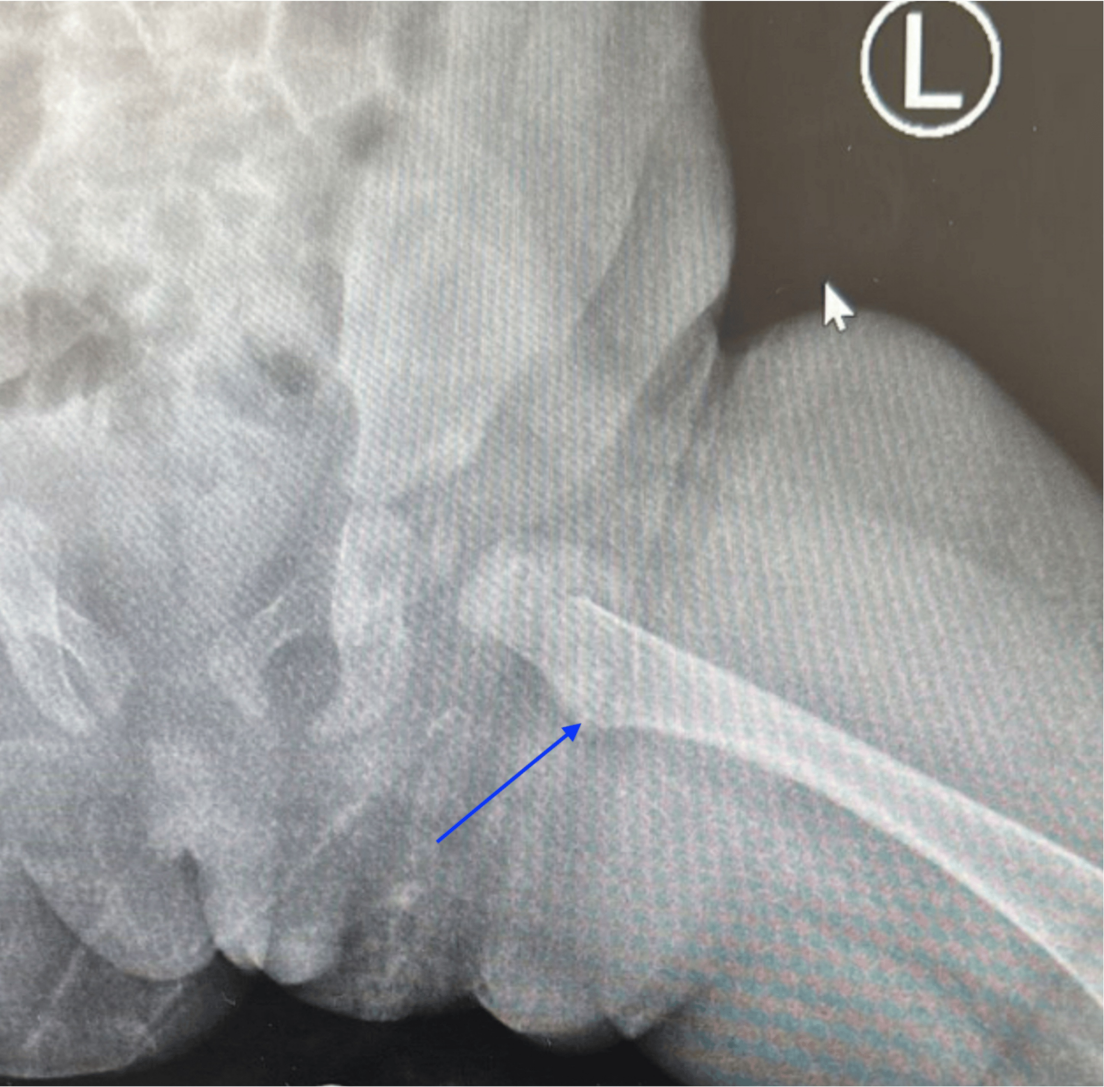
Proximal left femoral intertrochanteric fracture on radiograph of the patient.
